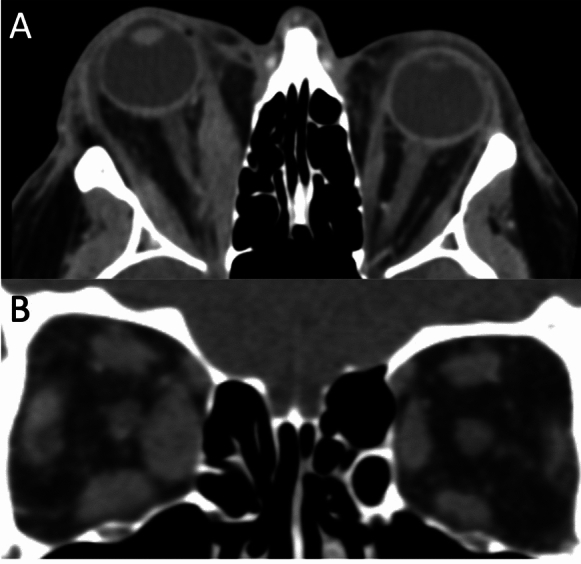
A Axial CT demonstrating right axial proptosis and medial rectus enlargement. B Coronal CT showing enlargement of the right extraocular muscles, with preserved left extraocular muscles
